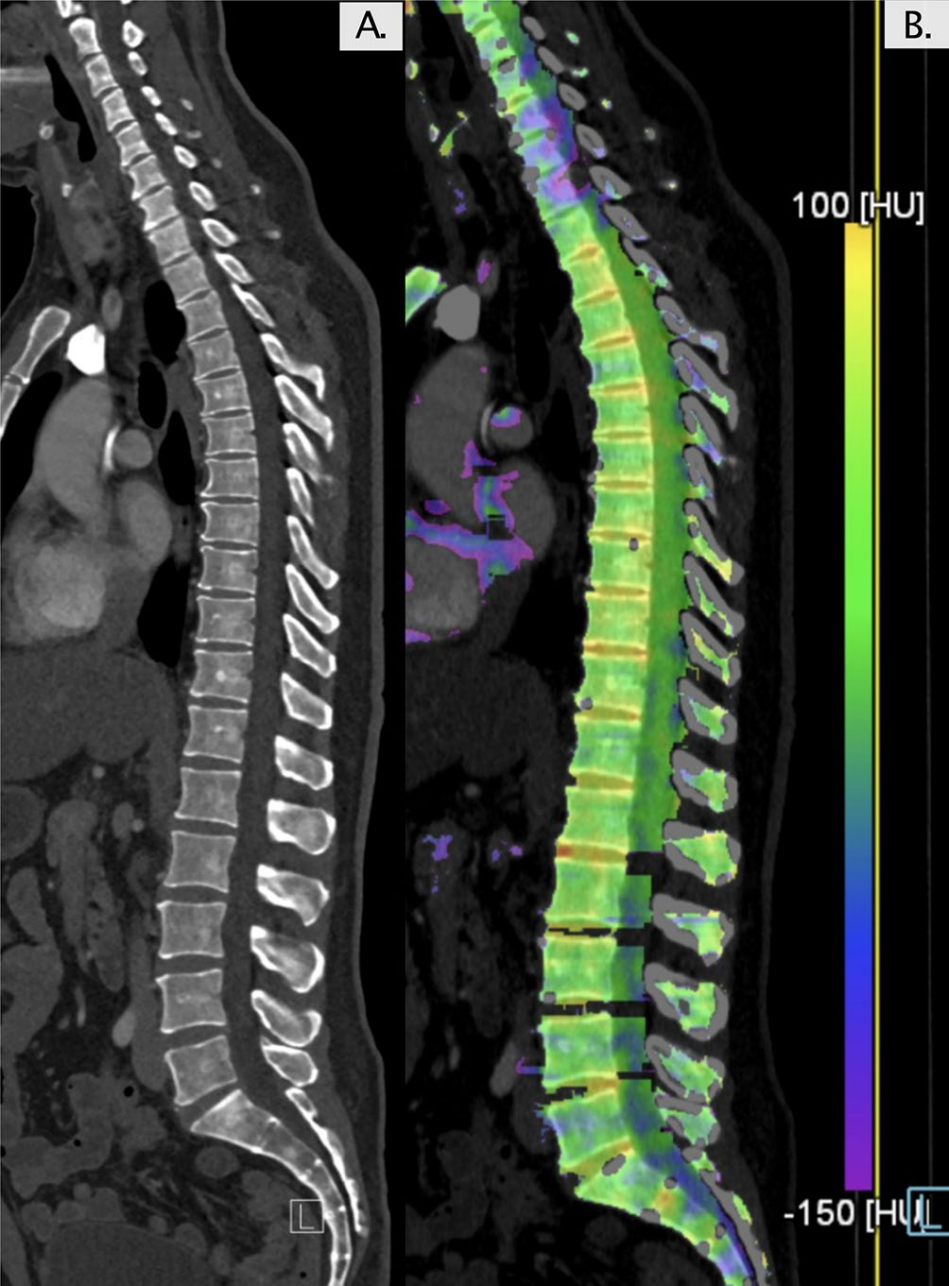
(A) Patient #8 with MCL showing normal bone structures on computer tomography (CT). (B) Dual-energy CT revealed elevated virtual-non-calcium values with diffuse edema of the bone marrow. The high bone marrow mast cell infiltration (70%) and high serum tryptase levels (885 µg/L) indicates a high mast cell disease burden.
